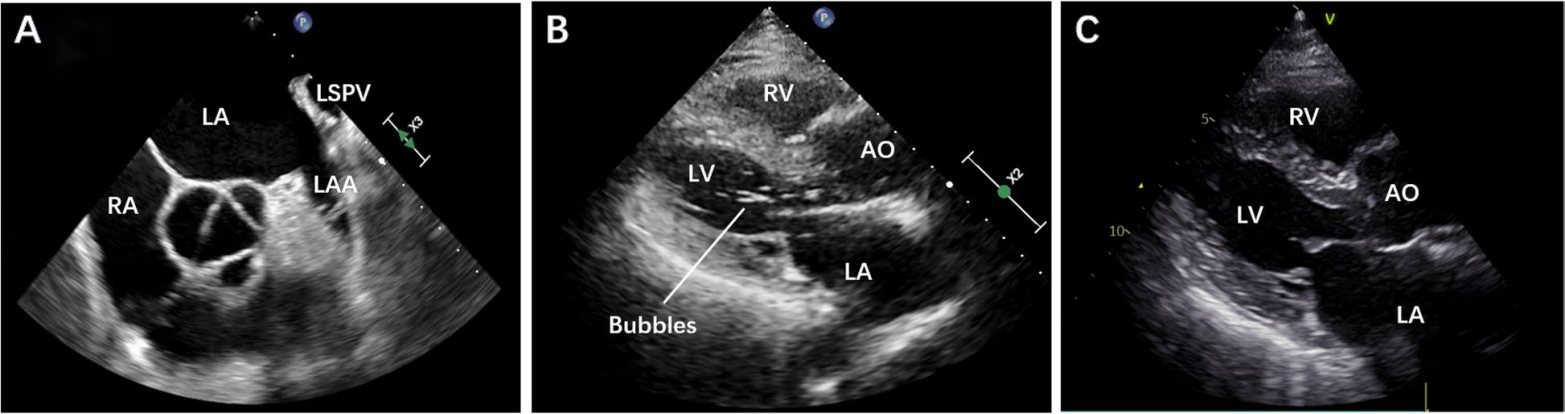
Preoperative transoesophageal echocardiography and intraoperative and postoperative transthoracic echocardiography. A Normal ostium of the LSPV on preoperative transoesophageal echocardiography. B Bubbles in the left heart on intraoperative transthoracic echocardiography. C No bubbles in the left heart on postoperative transthoracic echocardiography. LSPV = left superior pulmonary vein; LA = left atrium; RA = right atrium; LAA = left atrial appendage; RV = right ventricle; LV = left ventricle; AO = aorta
